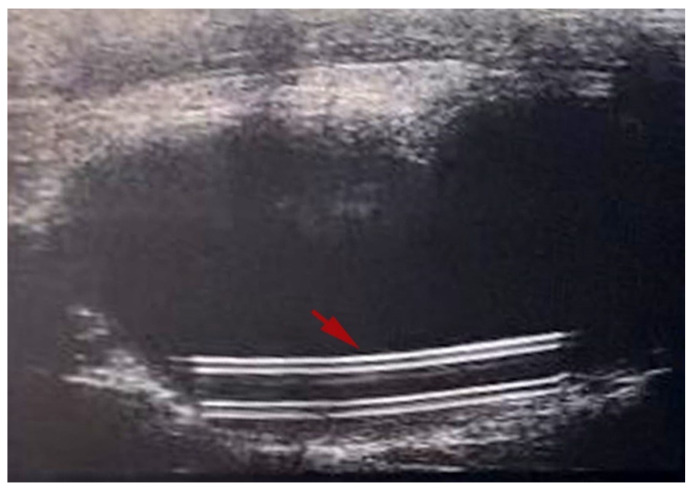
Minimally invasive monitoring made by ultrasound showing the urinary bladder at day 4. The catheter, indicated by the red arrow, appeared well positioned in situ.
